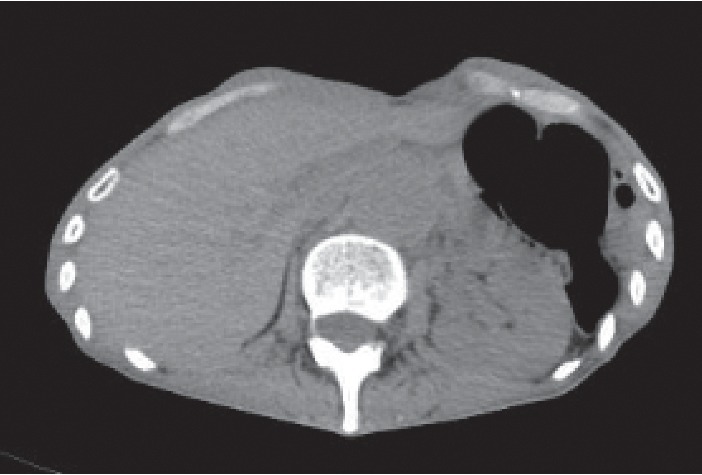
The computed tomography image does not show a distended stomach.
